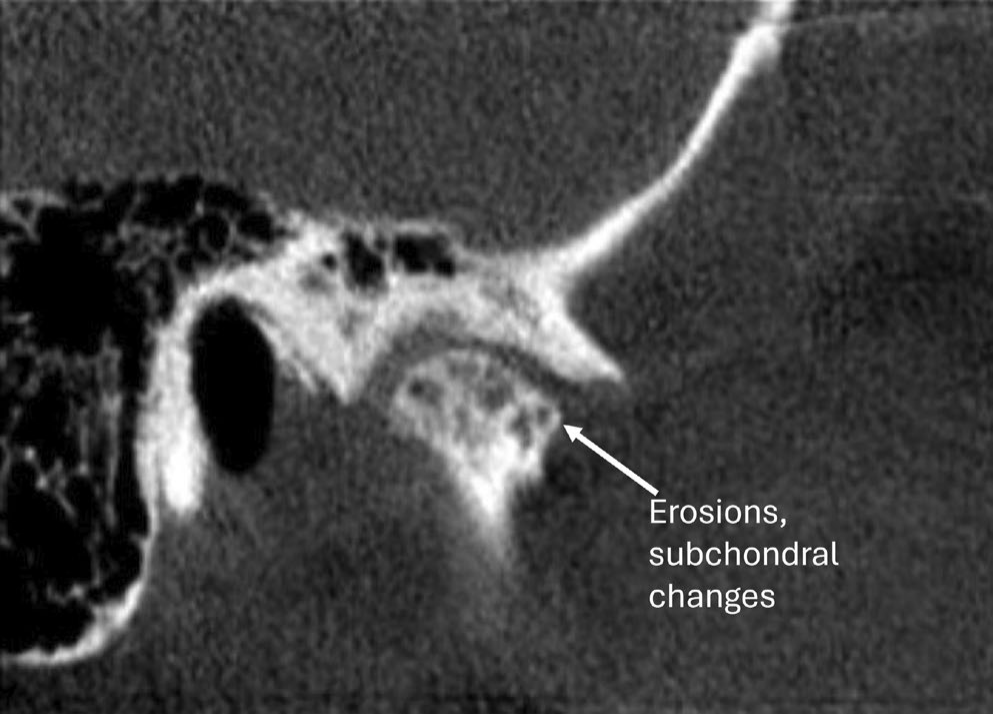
Sagittal CBCT view of left TMJ with degenerative changes. Sagittal view showing condylar erosion and subchondral changes in the articular eminence of the left TMJ.
